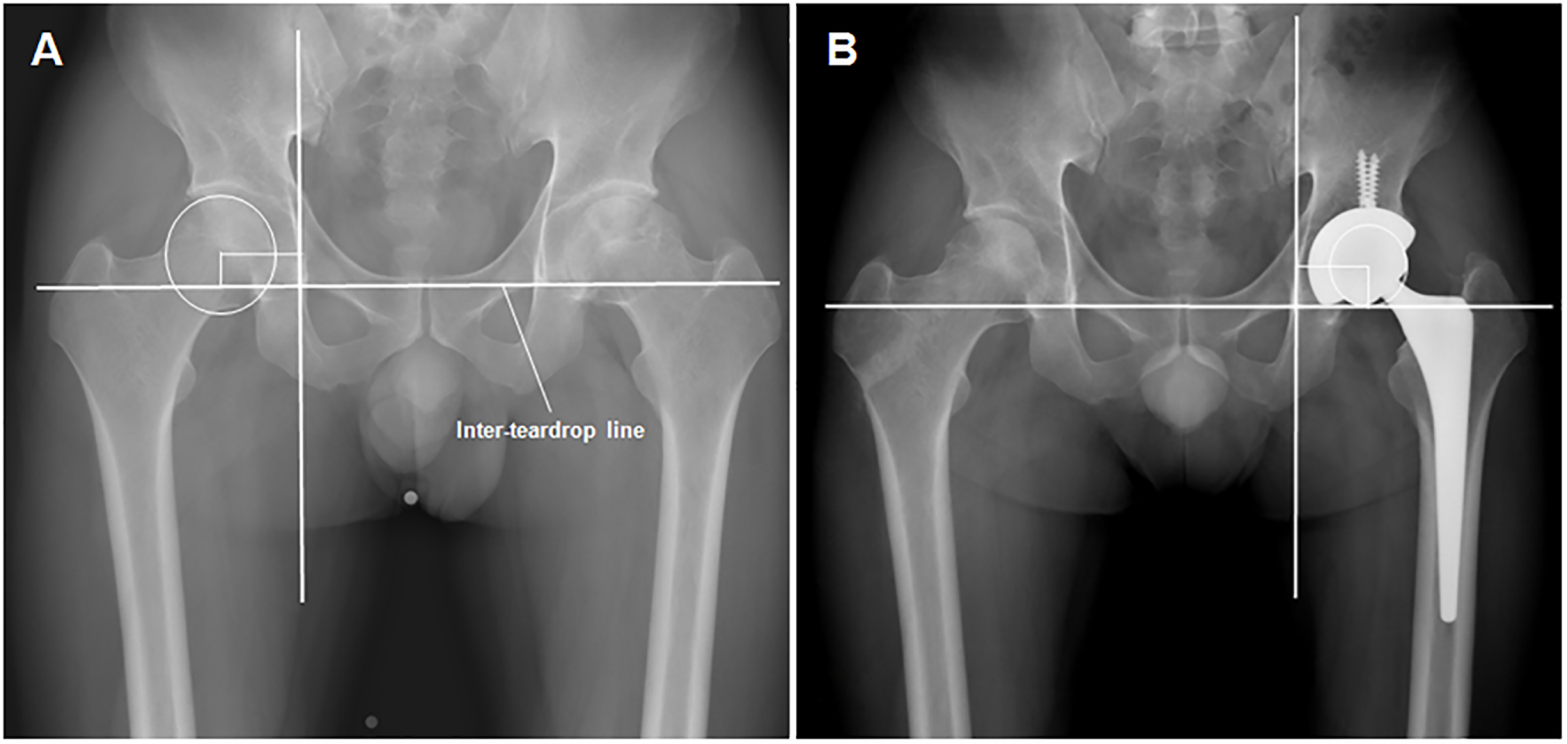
Pre- and postoperative anteroposterior pelvic radiographs of a 46-year-old male patient with osteonecrosis of the femoral head. (A) Preoperative anteroposterior template radiograph of the pelvis and (B) postoperative anteroposterior radiograph in a 46-year-old male patient with osteonecrosis of the femoral head show the radiographic measurements of the hip center of rotation (COR) and cup orientation. The inter-teardrop line is drawn between the floors of the acetabular teardrops. The actual measurements for determining the COR position are made on the unaffected side of the hip using a 10-cm standard calibration marker preoperatively (A), and on the reconstructed hip using the implanted prosthetic head postoperatively (B). The vertical height of the COR is measured as the distance between the (A) pre- or (B) postoperative COR and the inter-teardrop line. The horizontal distance of the COR is measured as the distance from the (A) pre- or (B) postoperative COR to the floor of the acetabular teardrop. The angle of inclination is the angle between the long axis of the opening ellipse and the inter-teardrop line. The angle of anteversion is calculated using an inverse sinus function (= arcsin (short axis/long axis)).
